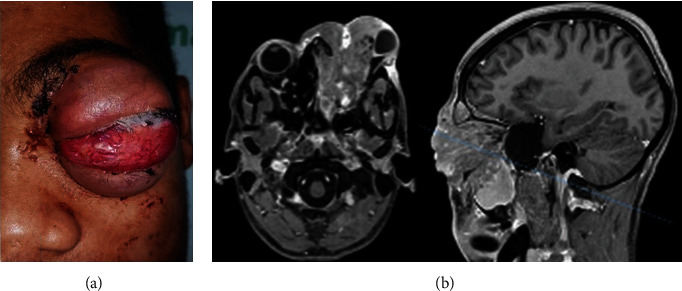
Clinical and imaging features. (a) Extraoral examination showed exophytic and expansive lesion around orbital region. (b) MRI axial and sagittal slices demonstrated tumor invasiveness into the periorbital region, sinonasal tract, left maxilla, and orbit roof.
